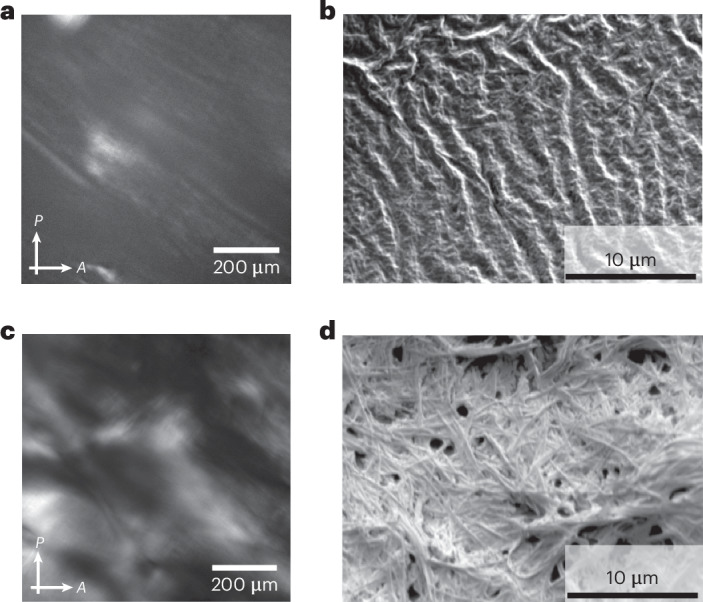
Forging by magnetic alignment within an MRI scanner. a,b, A cross-polarized optical image (a) and SEM image (b) for a system containing l,d-2NapFF in the presence of urea, urease and GdL allowed to evolve overnight in an MRI magnet. The vectors labelled P and A are the polarizer and analyzer axes, respectively. c,d, A cross-polarized optical image (c) and SEM image (d) for a system containing l,d-2NapFF in the presence of urea, urease and GdL allowed to evolve overnight outside of the magnet. Note that all the samples were kept in the same room with controlled humidity and temperature. In all cases, [l,d-2NapFF] = 5 mg ml^−1^, [urea] = 0.04 M, [urease] = 0.4 mg ml^−1^ and [GdL] = 14.3 mg ml^−1^.
